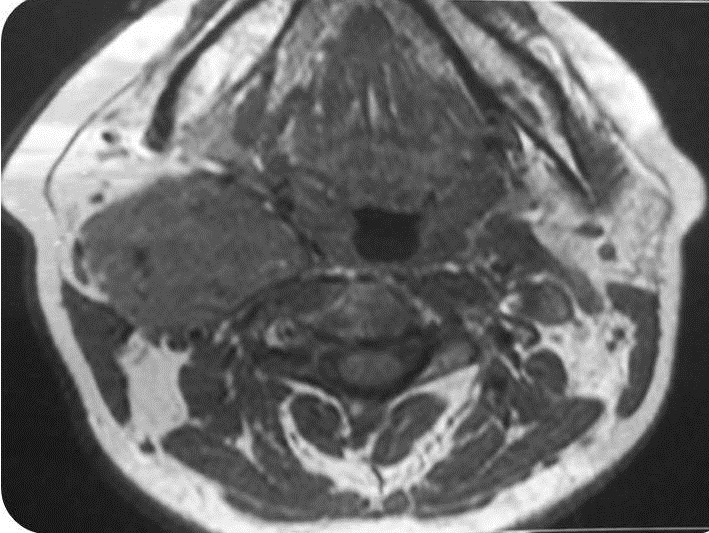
Cervical MRI; limited mass, iso intense in T1 sequence
